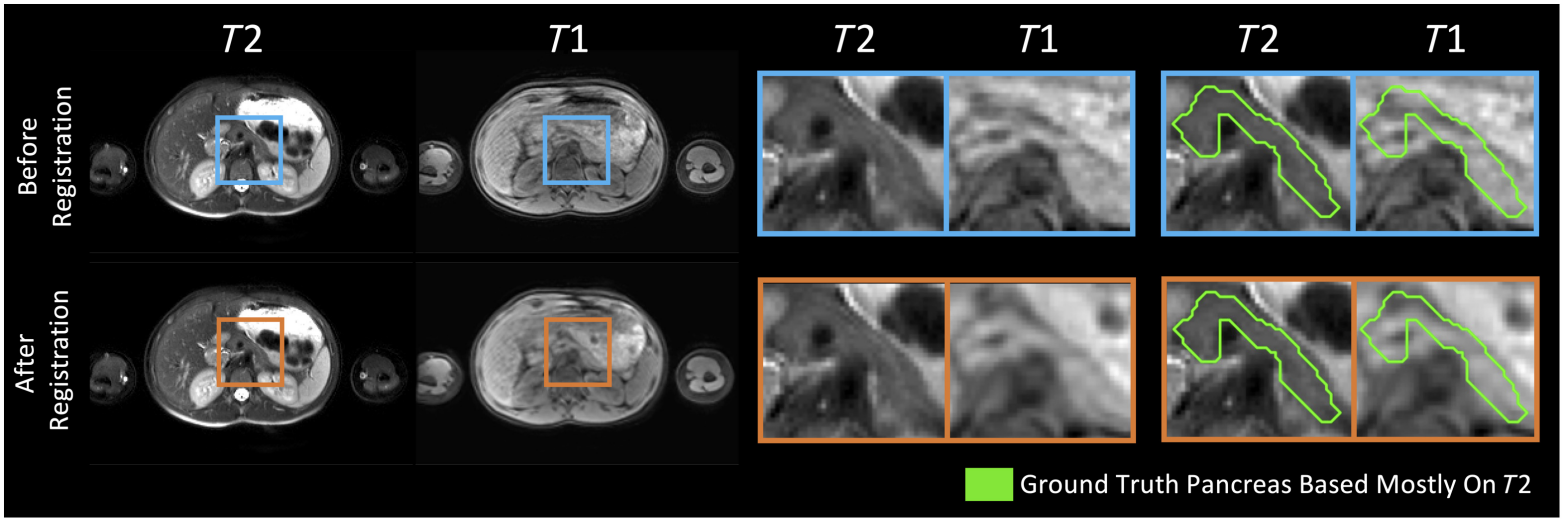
Our pancreas labels were mainly drawn based on the T2w MR images. Despite the use of effective deformable registration, the pancreas labels did not always line up well with the corresponding anatomy in the paired T1w MR images from the same subject and same imaging session. For example, the T1w image shown exhibits poor alignment with both the T2w image and the pancreas mask, even after registration (bottom right). Persisting image misalignment makes it unclear how best to fuse T1w information to see gains in segmentation performance. The example shown exhibits particularly poor alignment.
